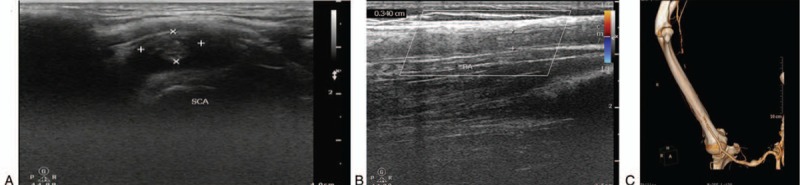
(A) Thrombosis of the right subclavian artery distant with stenosis (diameter stenosis rate was 77.1%). (B) Poor filling of local blood in the right brachial artery, thrombosis was not excluded. (C) CTA of the right upper limb: aneurysm occurred in the right subclavian, thrombosis of the right brachial artery medium-to-distal. CTA = computed tomography angiography.
